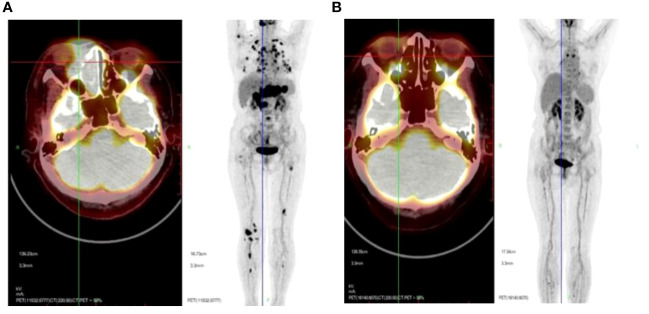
Representative PET/CT scans presented to offer a comparative evaluation of a patient diagnosed with stage IV ENKTL before (A) and after (B) treatment, with the assessment of therapeutic outcome as CR.
